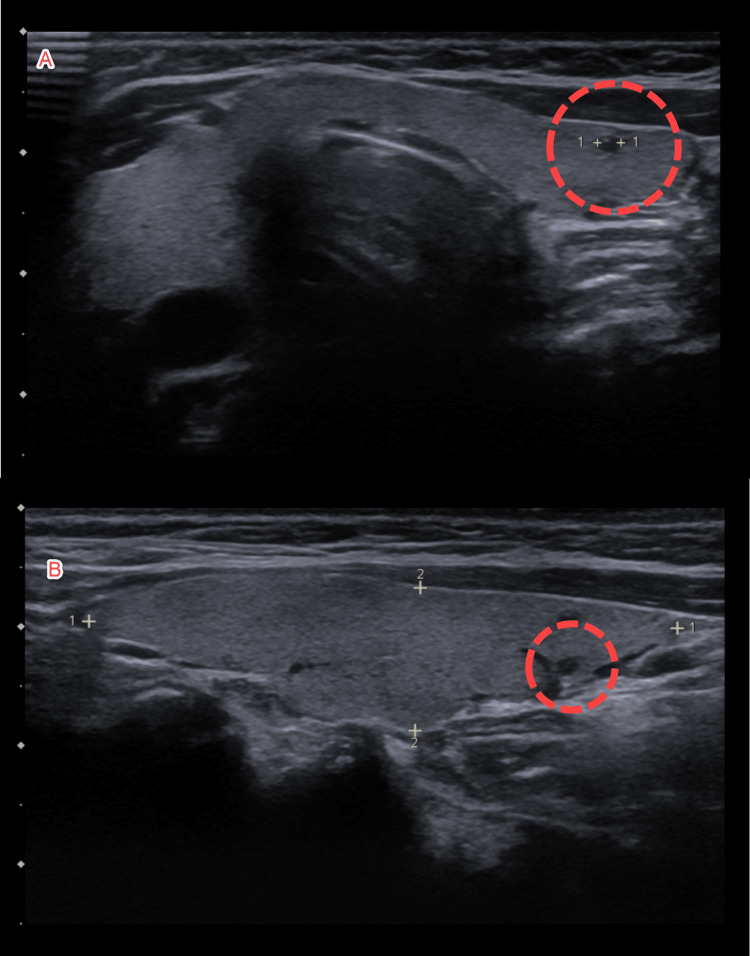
Thyroid ultrasound showing two nodules in the left upper pole (A and B)
